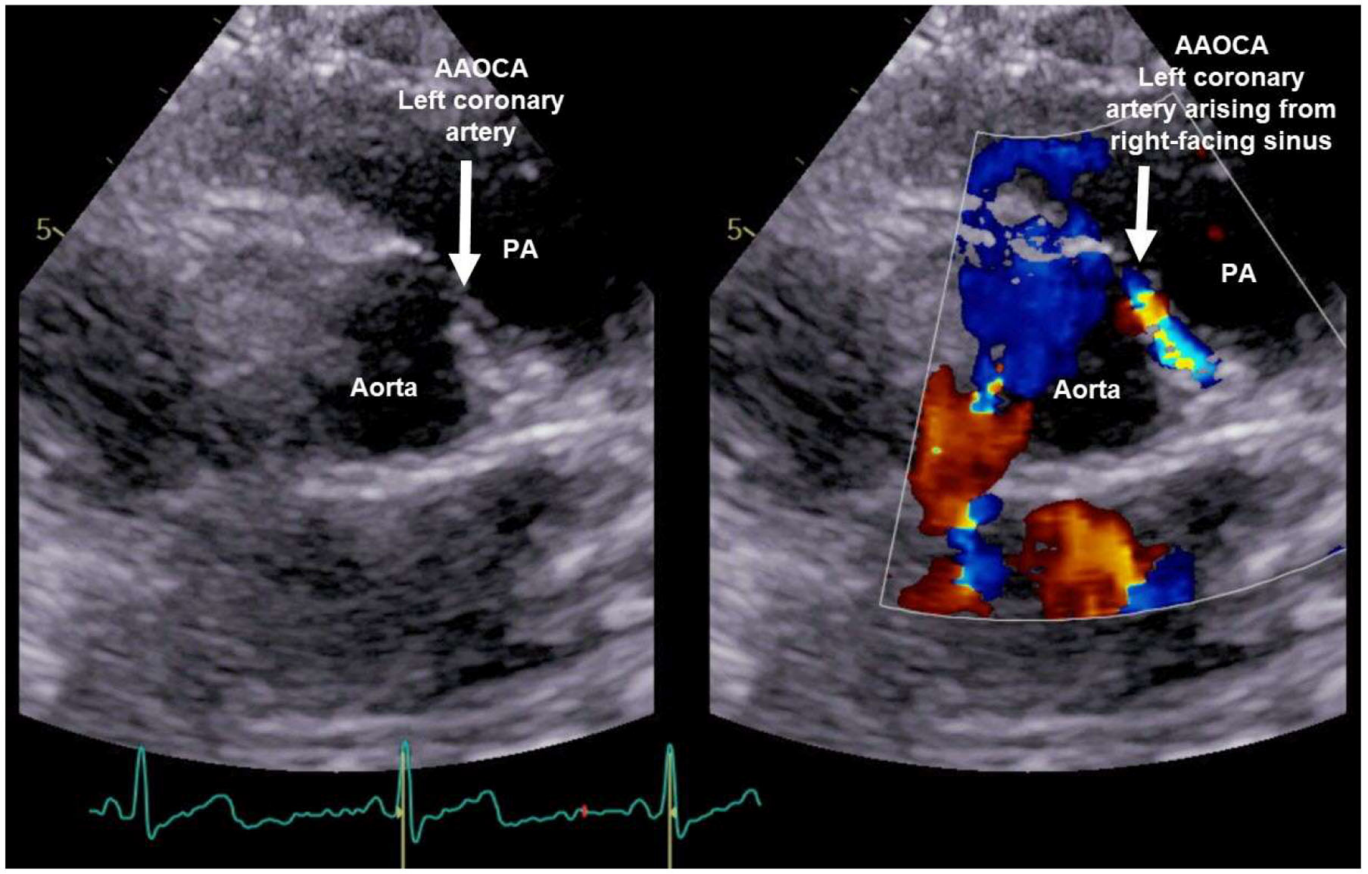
Echocardiography of patient 1: Coronary flow pattern arising from the right-facing sinus and continuing between aorta and pulmonary artery (PA) suggesting an interarterial/intramural course of the left coronary artery.
